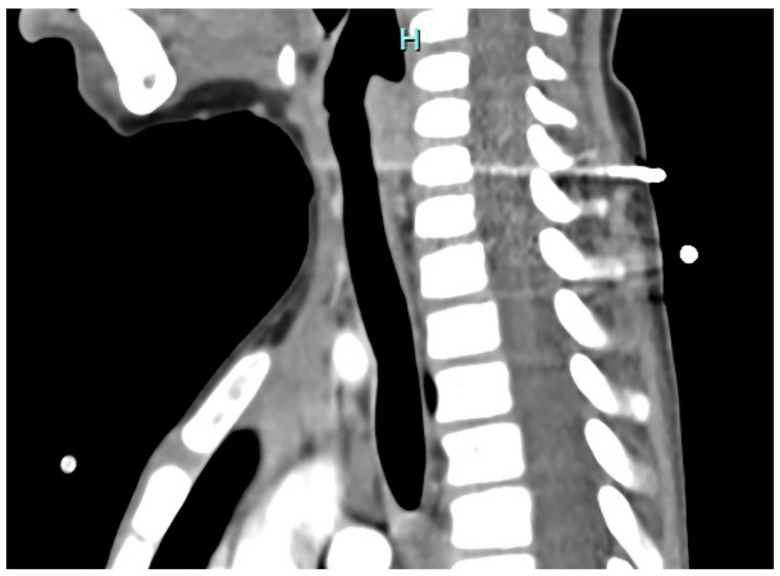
Case 2. Neck and chest CT scan showing preserved tracheal patency. H = upper.
